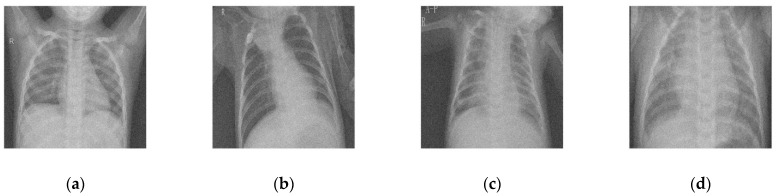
COVID-19 noisy CT image dataset corresponding to Figure 2; (a) Noisy CT1 image; (b) Noisy CT2 image; (c) Noisy CT3 image; (d) Noisy CT4 image.
